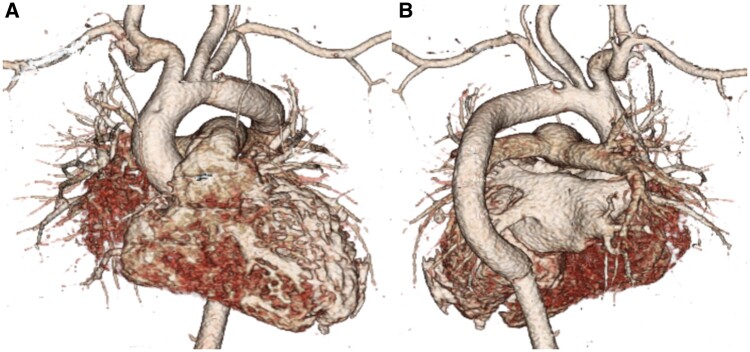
Postoperative computed tomography showing widely patent graft. (A) Anterior view and (B) right posterior oblique view.
